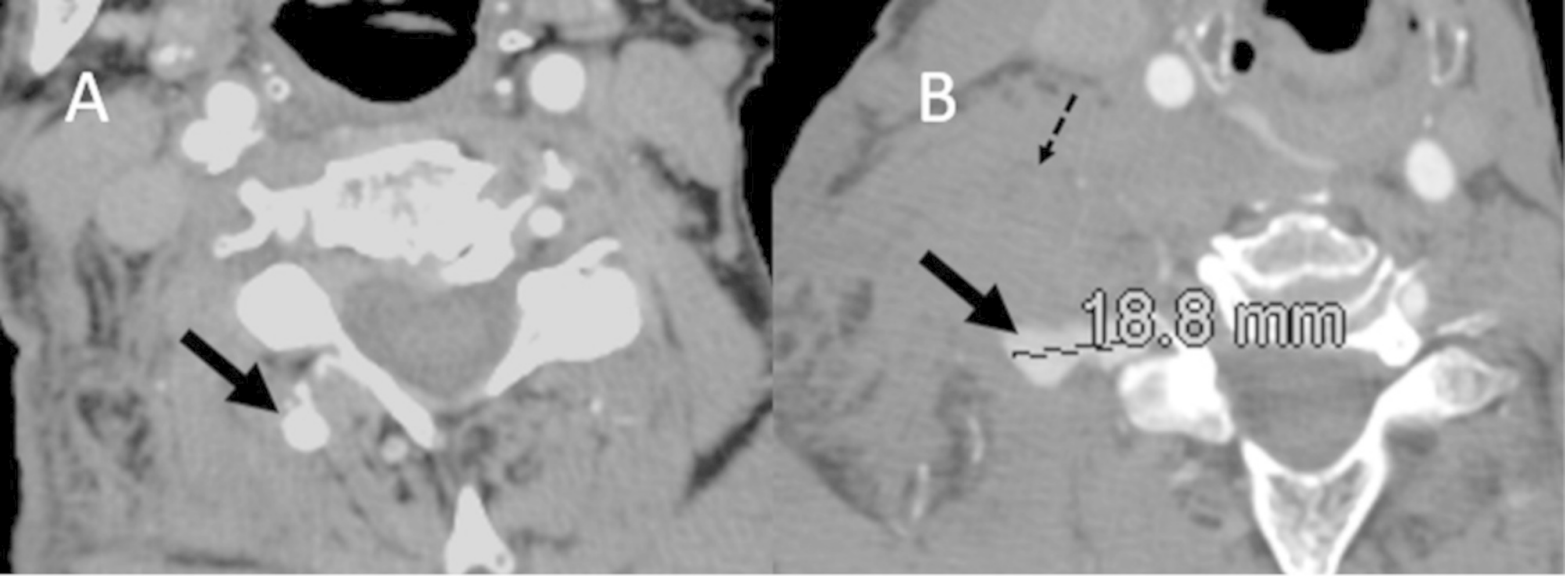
(A) Axial section CT angiography demonstrating a focal prominence at the distal aspect of the ascending cervical artery (black arrow). (B) Axial section CT angiography demonstrating extravascular contrast deep within the right lateral neck in conjunction with a probable right lateral neck hematoma (dotted arrow).
